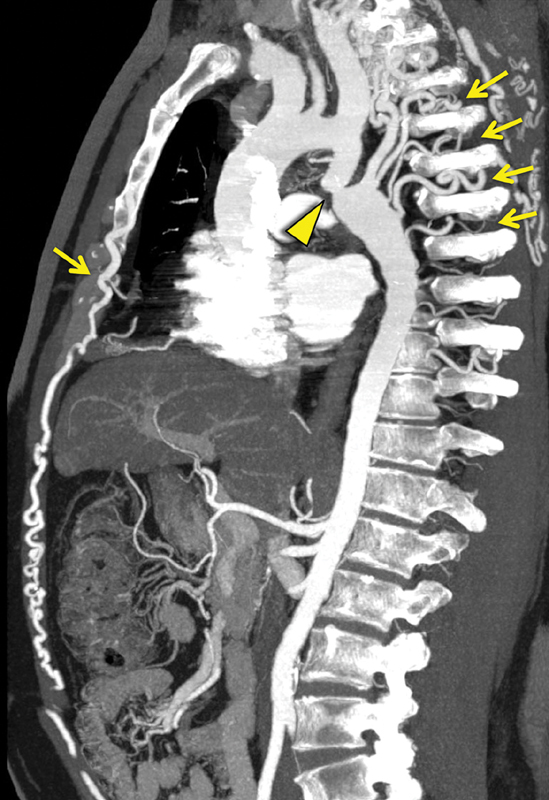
Multidetector computed tomography angiography sagittal reconstruction demonstrating the presence of aortic coarctation at the level of isthmus proximal third of thoracic aorta (arrowhead) with hypertrophic compensatory collateral arterial circulation from the intercostal and mammary arteries (arrows).
